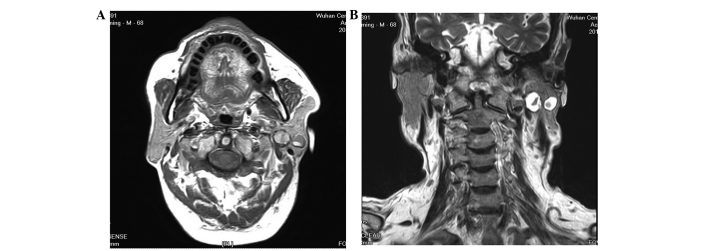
(A) Axial T1-weighted magnetic resonance imaging showing two independent masses in the superficial and deep lobes of the parotid gland on the left side. The two tumors were well-marginated. (B) Coronal T2-weighted magnetic resonance imaging showing moderate enhancement in the peripheral component and signal hypointensity in the central component of the two tumors.
